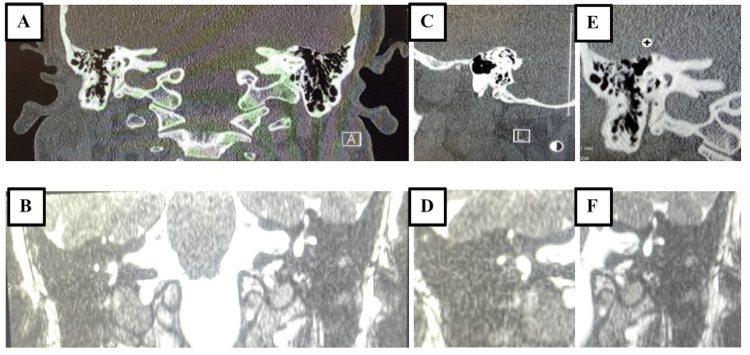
Bilateral superior semicircular canal dehiscence shown in coronal CT (A) and MRI (B). Right side zoom: CT (C), MRI (D). Left side zoom: CT (E), MRI (F).
